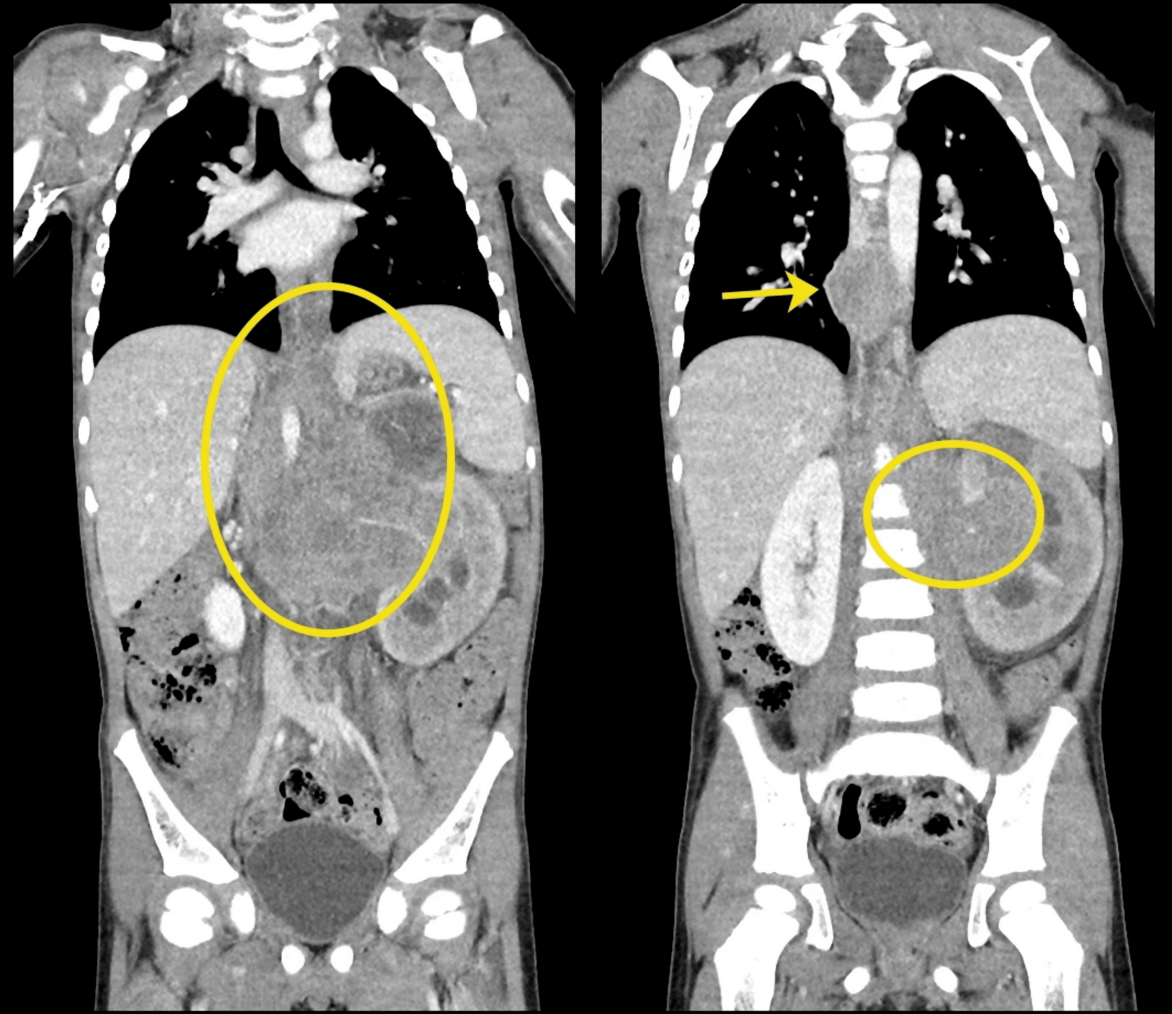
Coronal Views of CT Chest/Abdomen/Pelvis The irregular mass is seen in these coronal CT views (circled). The thoracic mediastinal lymphadenopathy is also visualized (arrow).
